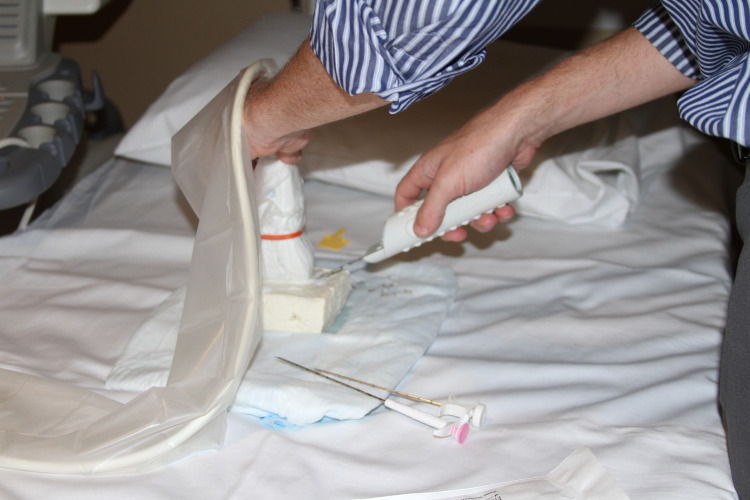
A resident using the tofu and blueberry model to practice ultrasound-guided breast biopsies.
